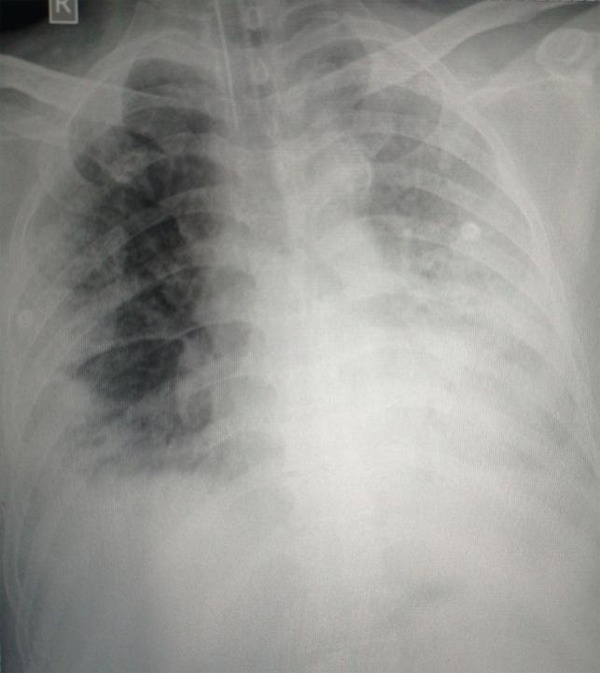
Bedside Portable Chest X-Ray Showing Bilateral Diffuse Lung Filed Infiltration.
